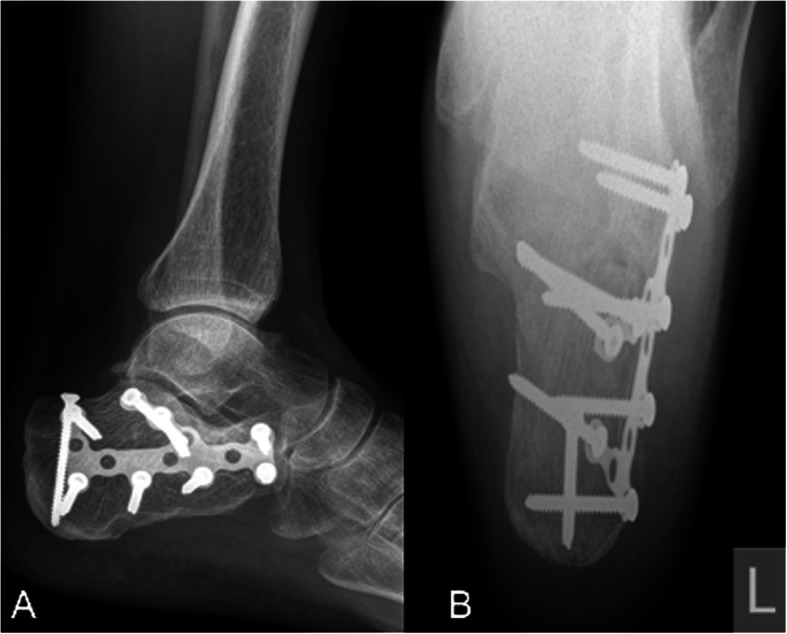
Case 1 Postoperative radiographs. Lateral (A) and axial (B) radiographs after ORIF via an extended lateral approach
